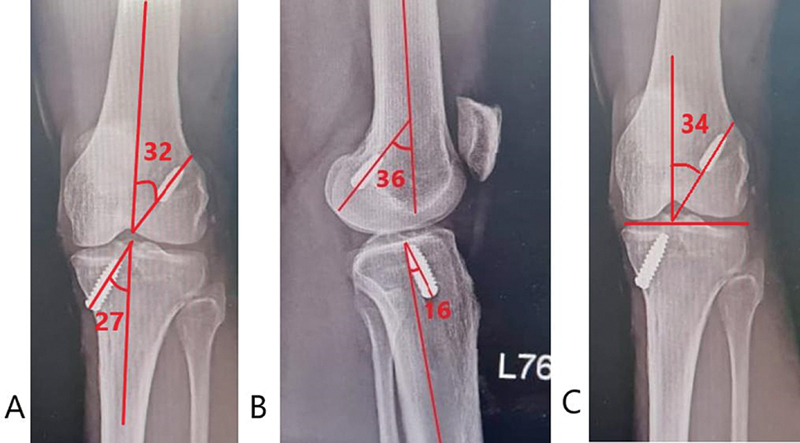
Radiological assessment of tunnel angles in postoperative x-rays. A) Coronal femoral and tibial tunnel angles. B) Sagittal femoral and tibial tunnel angles. C) Graft inclination angle.
